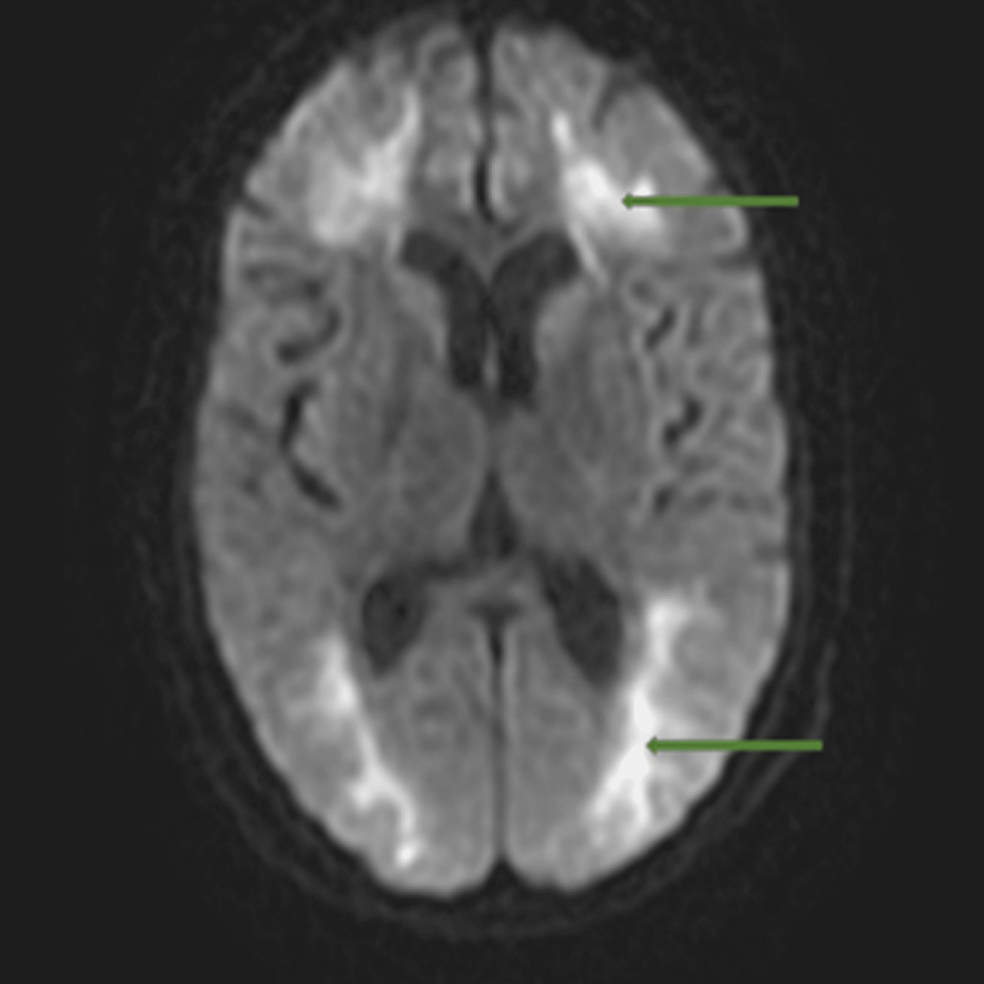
Magnetic resonance imaging (MRI) AX DWI showing diffuse bilateral cerebral white matter changes (green arrows) involving the frontal, parietal, occipital, and temporal lobes.
